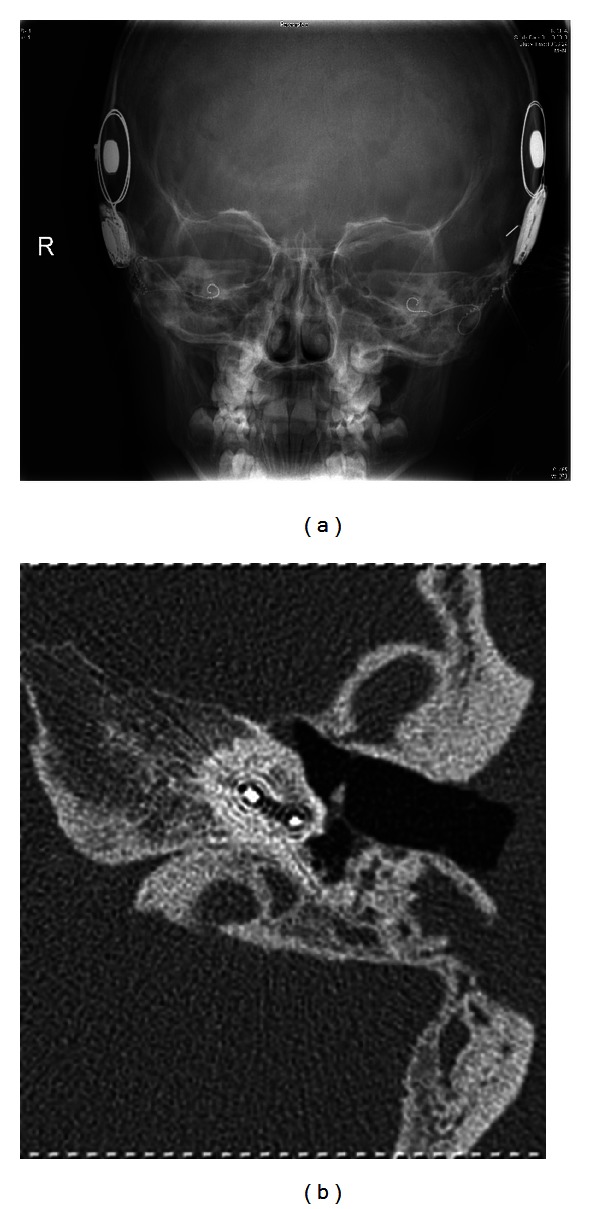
(a) Conventional X-ray cochlear view, (b) conventional multislice computed tomography.
